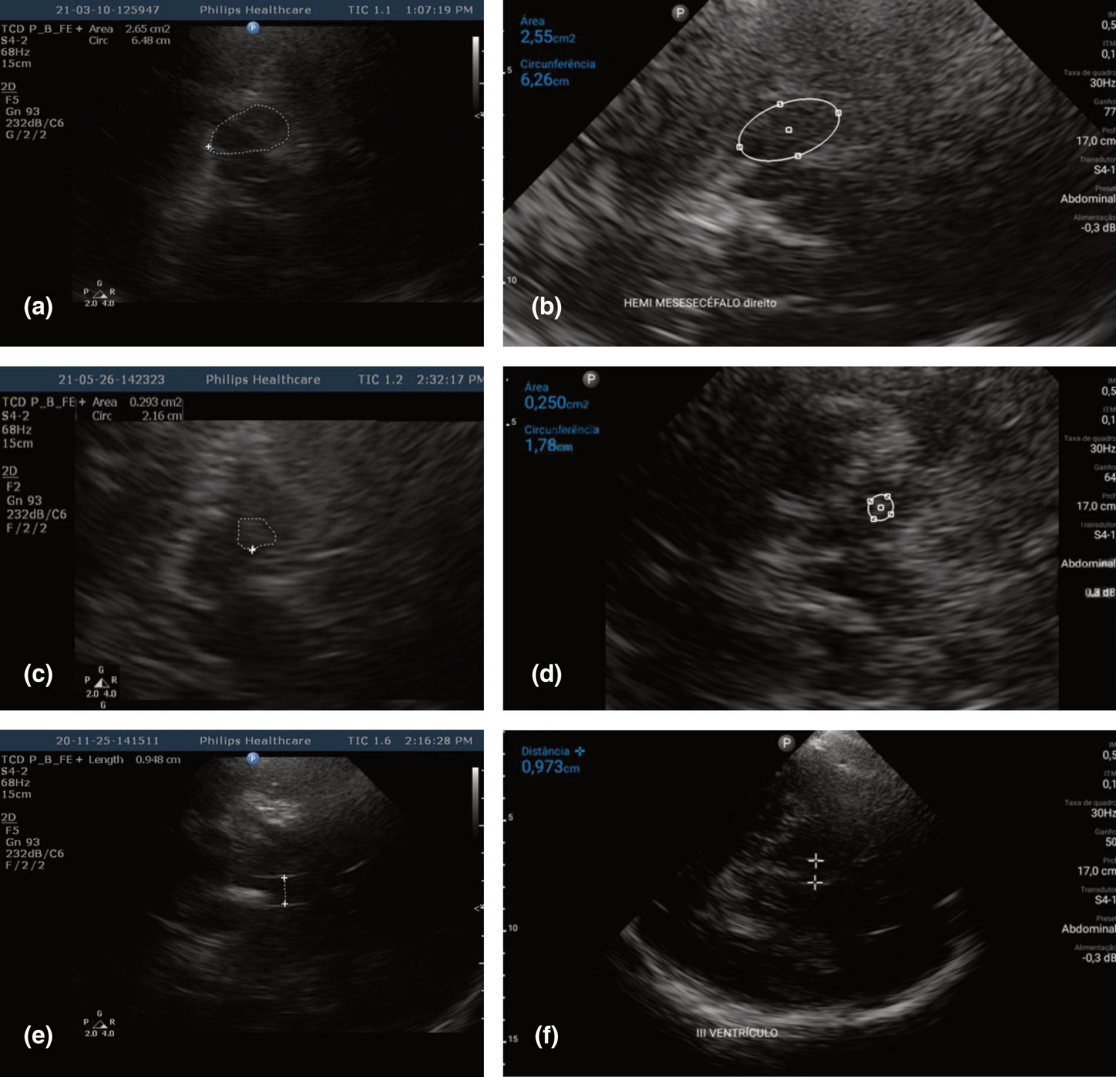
Axial transcranial ultrasound images of the same patient with Parkinson's disease show similar values of measurements acquired by the two US systems. Left row images (A‐C‐E) acquired with traditional equipment. The images of the right line (B‐D‐F) were acquired with the handheld US. (A) TCS in traditional equipment shows the manual delimitation of the right hemisencephalon to calculate the area. (B) TCS on handheld US shows that the right hemisencephalon area was calculated by an ellipse. (A and B) ‐ Measurements of the hemisencephalon area: 2.65 cm^2^ and 2.55 cm^2^. (C) TCS in traditional equipment shows that manual delimitation circumscribes the echogenic area of the left SN. (D) TCS on handheld US shows that the echogenic area of the left SN was calculated by an ellipse. (C and D) – The echogenic area of the ipsilateral substantia nigra (SN): 0.293 cm^2^ and 0.250 cm^2^. (E) TCS in traditional equipment shows the measurement of the third ventricle. (F) Handheld US TCS shows the measurement of the third ventricle. (E and F) Diameter of the 3rd transverse ventricle: 0.948 cm and 0.973 cm.
